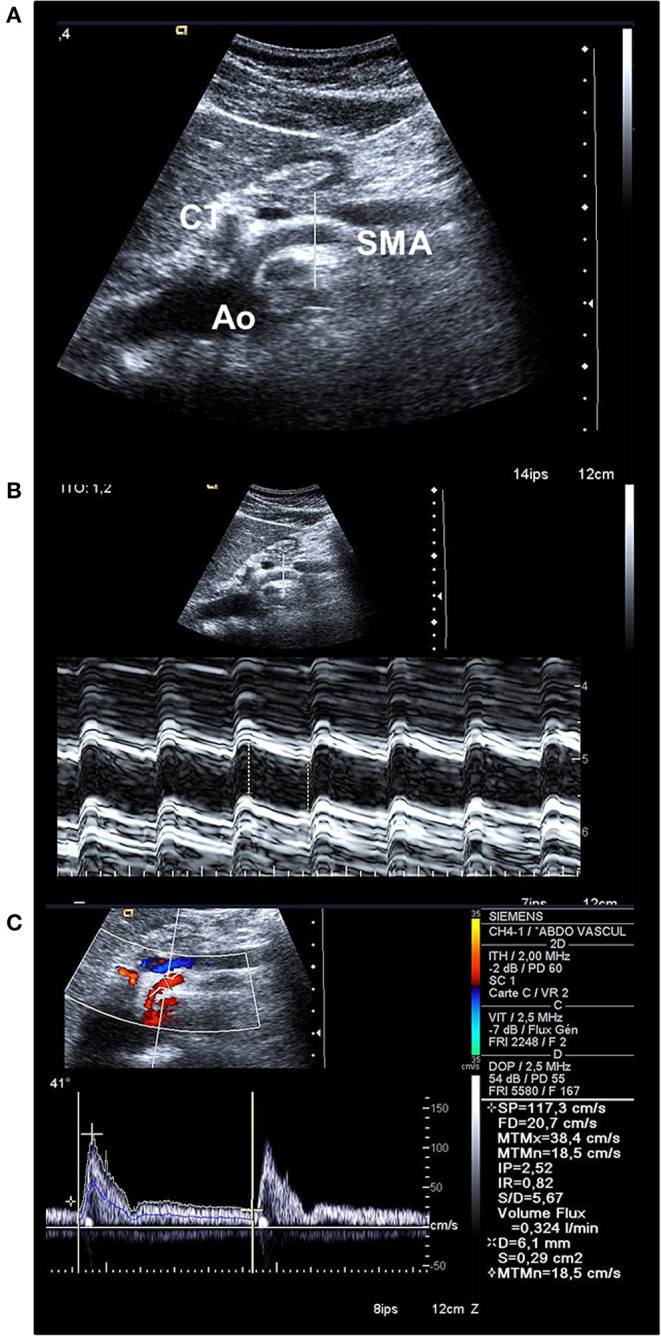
(A) Bidimensional longitudinal cross-sectional view of the aorta (Ao), the coeliac trunk (CT), and the SMA permitting to place the line for M-mode strictly orthogonal to the longitudinal axis of the artery. (B) M-mode activation on the SMA permits to place calipers to measure systolic and diastolic inner diameters and to calculate the mean diameter (here, 0.61 cm). (C) Doppler spectral analysis of the blood flow velocity waveform recorded in the SMA; the blue line represents the instantaneous mean blood flow velocity (BFVel = 18.5 cm/s) obtained after application of the angle-correction between the Doppler beam and the longitudinal axis of the artery (41°). The BFVo) is then calculated (324 mL/min).
